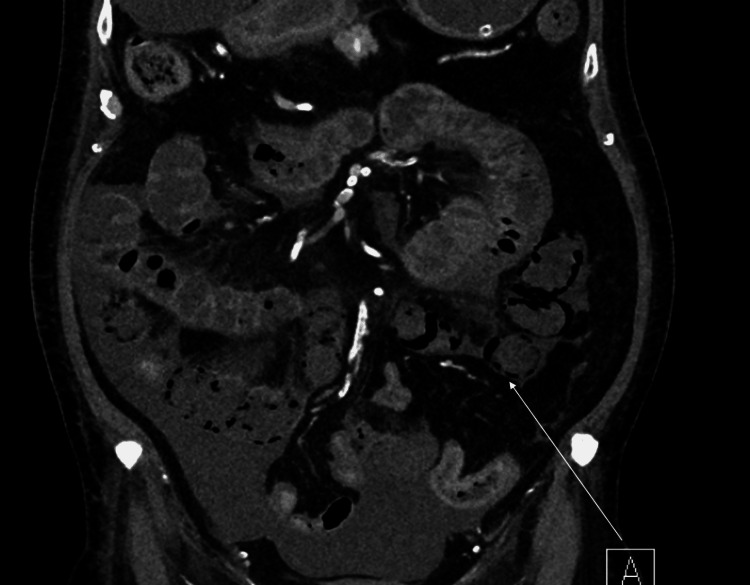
A coronal plane image of the CT abdomen and pelvis demonstrating jejunum and ileum wall hypoenhancement in comparison to the rest of the bowel, as well as pneumatosis intestinalis of jejunum and ileum as shown by the arrow.
